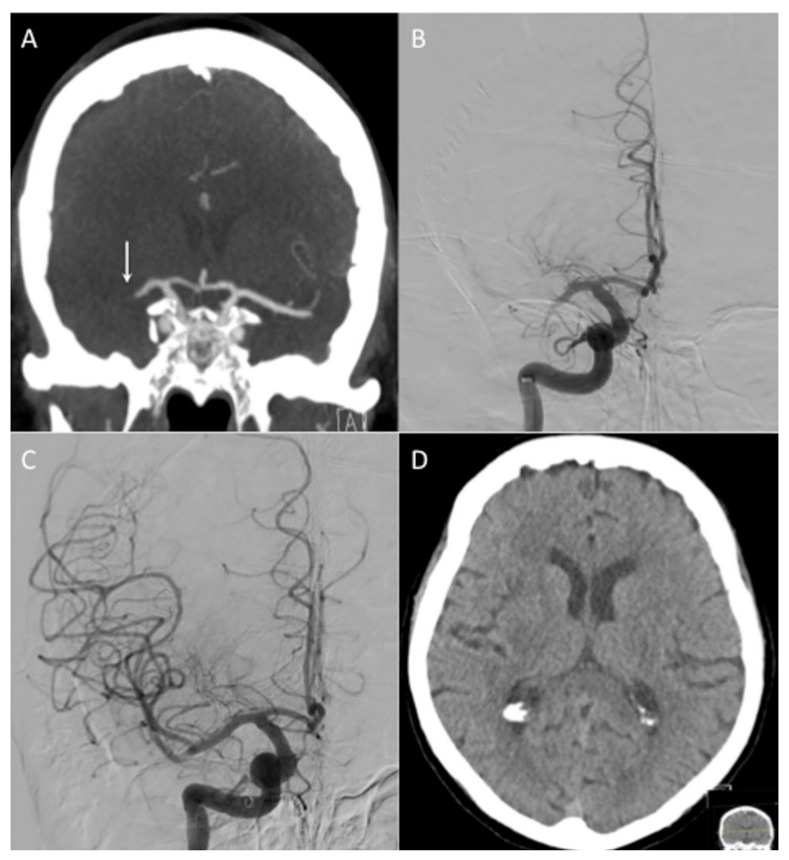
Patient 20 presented with an NIHSS of 12 and mid M1 occlusion on CT angiography (A, white arrow). After tracking a Ballast guide catheter (Balt Extrusion, Montmorency, France) into the high cervical ICA, angiography confirmed the clot (B). A Q6 catheter was ‘snaked’ to the clot face and aspiration thrombectomy was performed, resultingin TICI 3 recanalization in 1 pass (C). There was good preservation of grey-white matter differentiation on the 24-hCT scan with no evidence of haemorrhage (D). The groin puncture to recanalization time was 7 min.
